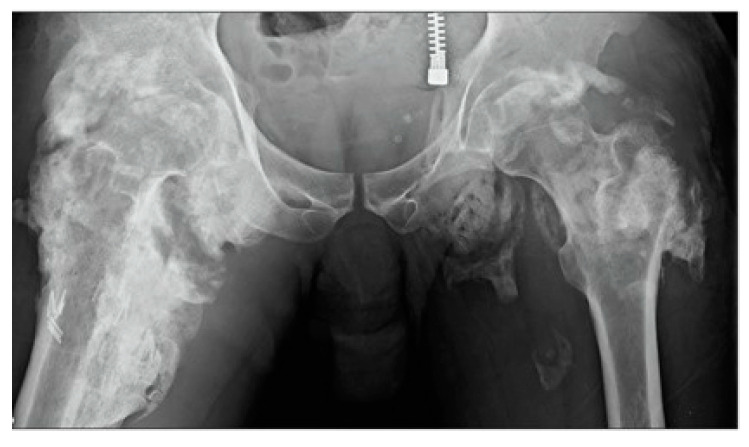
Radiographic-based diagnostic imaging represented massive bilateral peri-articular HO in an immobilized patient with SCI [118].
